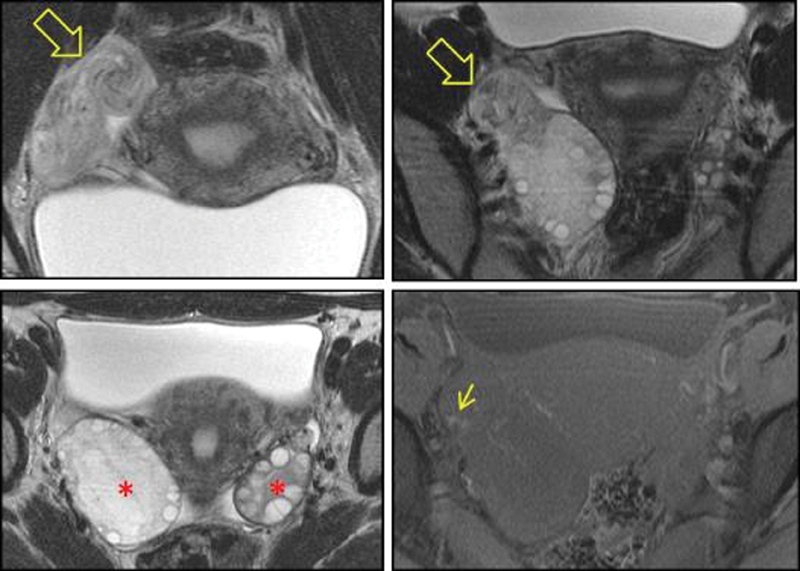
Magnetic resonance imaging evaluation: Coronal and Axial T2WI nicely demonstrates the twisting of the ovarian pedicle - the whirlpool sign (open arrow); the enlargement of the right ovary compared with the left one is also evident (asterisk); Fat Sat T1WI shows the presence of blood in the vascular pedicle in favor of vascular congestion (small arrow).
